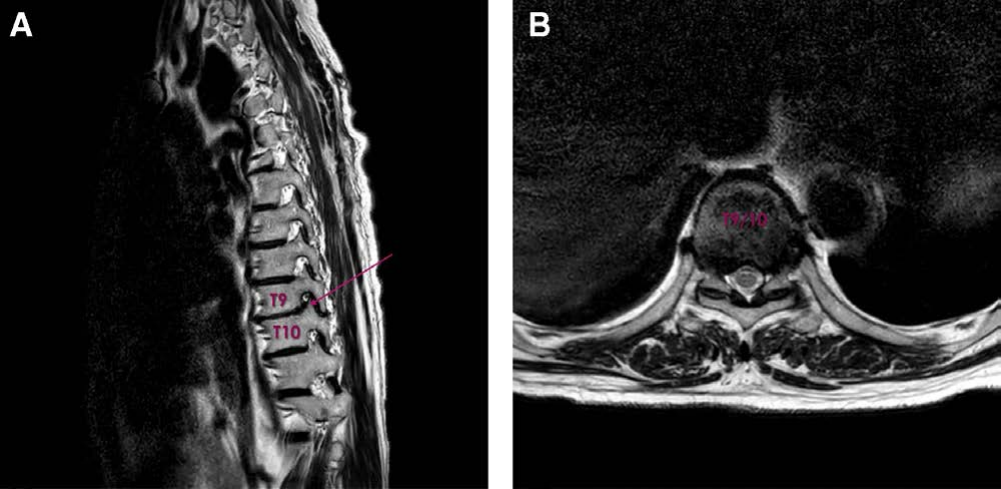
Magnetic resonance T2-weighted imaging of thoracic spine showing the foraminal herniated disc at the left T9/10 level. (A) Sagittal plane. (B) Axial plane.
